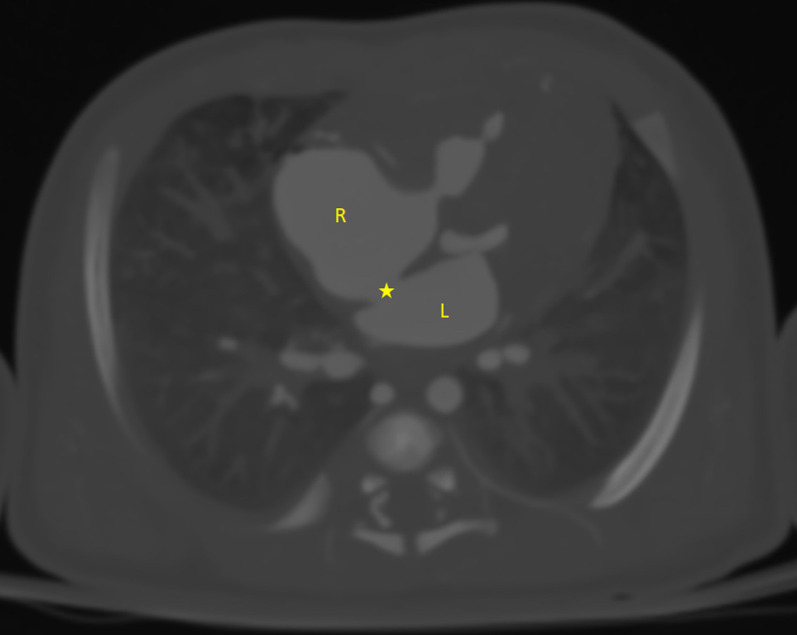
pedPMCTA in a 2-week-old male infant (weight: 3.1 kg), following sequential femoral arterial and then venous injections of lipophilic contrast agent. Axial contrast-enhanced CT image showing contrast passage from the right atrium (R) to the left atrium (L) through a patent foramen ovale (yellow star), suggesting intracardiac shunting. In this case, arterial enhancement was improved following venous injection, likely due to the presence of this communication. This observation highlights the impact of physiological postnatal shunts on vascular distribution in pedPMCTA
